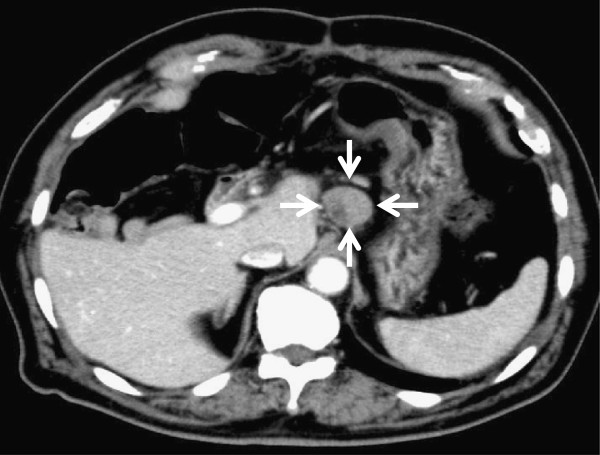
Postoperative contrast-enhanced computed tomography findings. Six months after surgery, a computed tomography examination revealed multiple swollen lymph nodes around the abdominal aorta. One of the para-aortic lymph nodes located at the left side of the caudate lobe is indicated by arrows.
